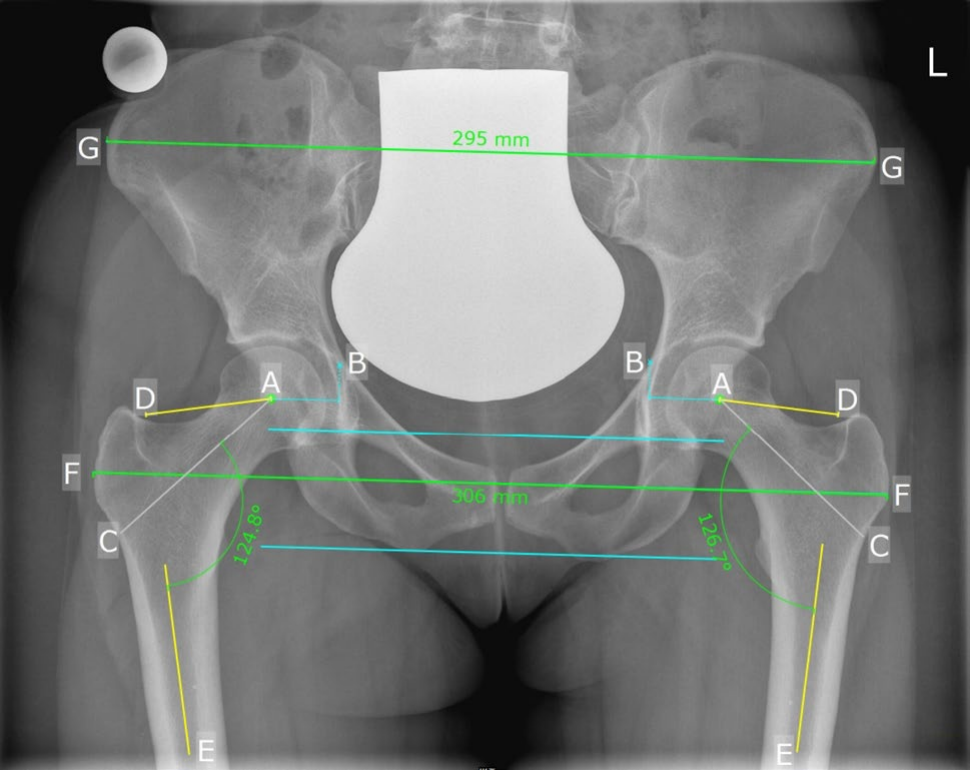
Measurements performed on a posteroanterior pelvic radiograph using reference ball (25 mm): femoral offset (AD) and acetabular offset (AB), CCD angle (between AC and CE), as well as intertrochanteric distance (FF) and pelvic width (GG)
